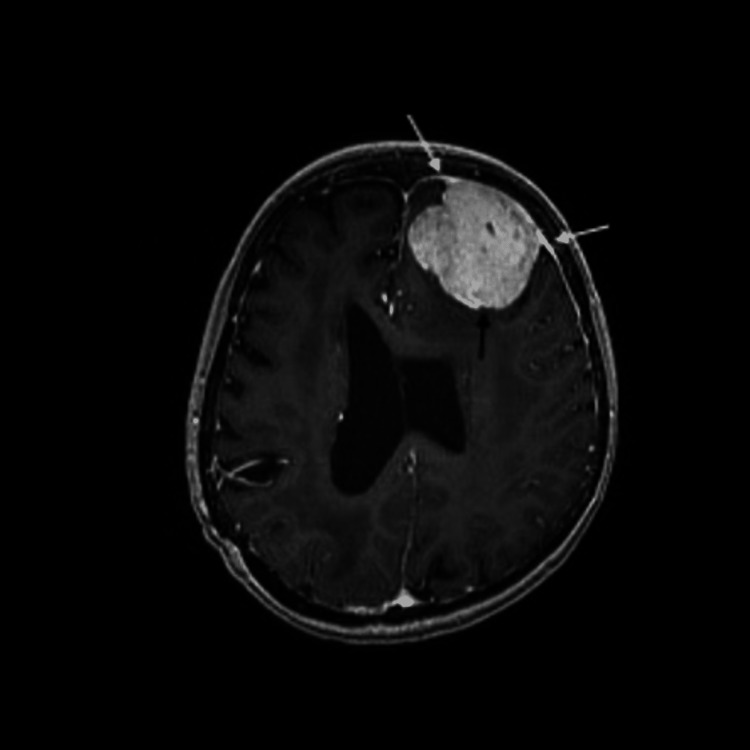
A T1 weighted post gadolinium axial MRI showing a 2.5cm, homogeneously enhancing meningioma (black arrow) with enhancing dural tails (white arrows).
